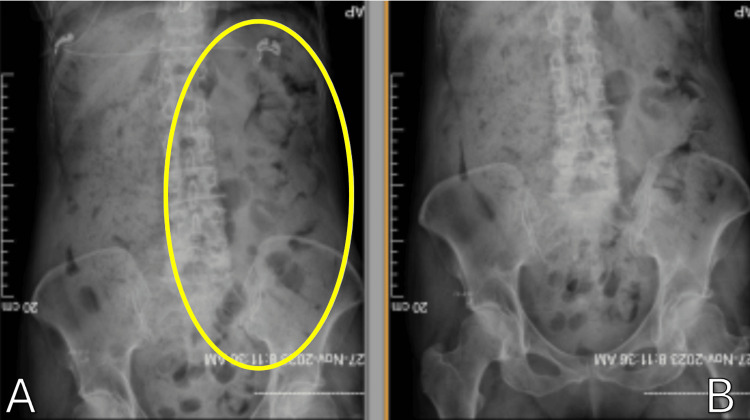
(A-B) Abdominal X-ray revealing heavy stool burden
